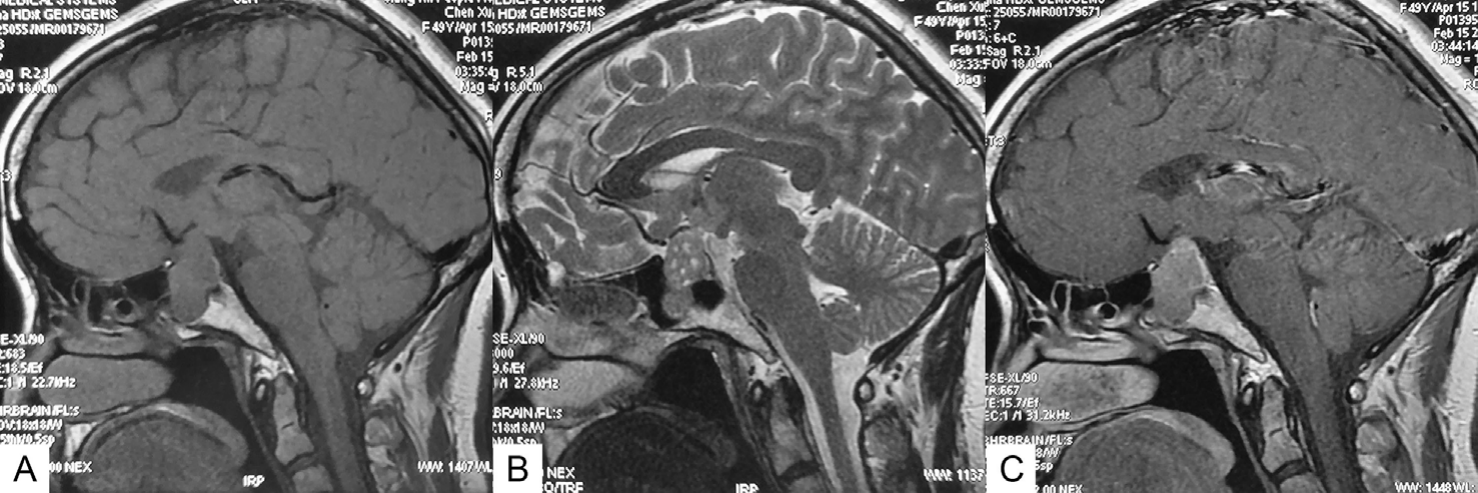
Sagittal MR images of an SCA with typical multiple microcysts. (A) T1-weighted image. (B) T2-weighted image, showing multiple microcysts. (C) Contrast-enhanced image.
